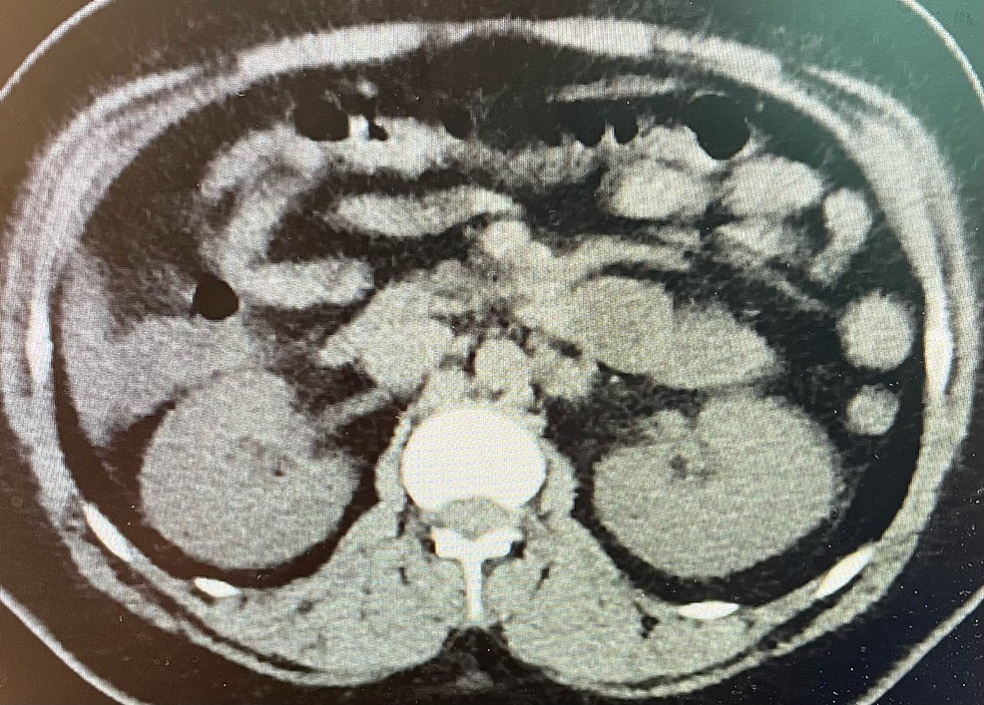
CT abdomen/pelvis with contrast demonstrating no acute pathology.
